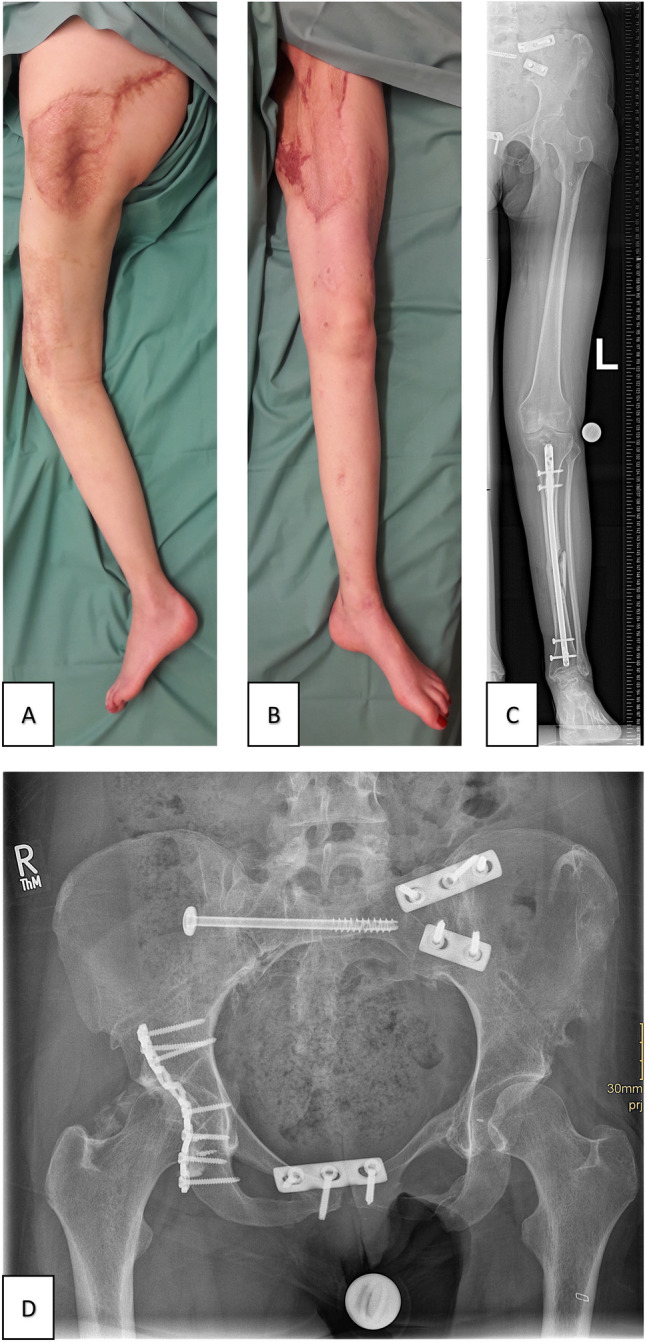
Patient 2 follow-up 18 months after the accident. A Clinical photographs of salvaged limb B Clinical photographs of salvaged limb, uneventful healing of the mesh graft. C Radiograph of the salvaged limb. D Radiograph of the pelvis
